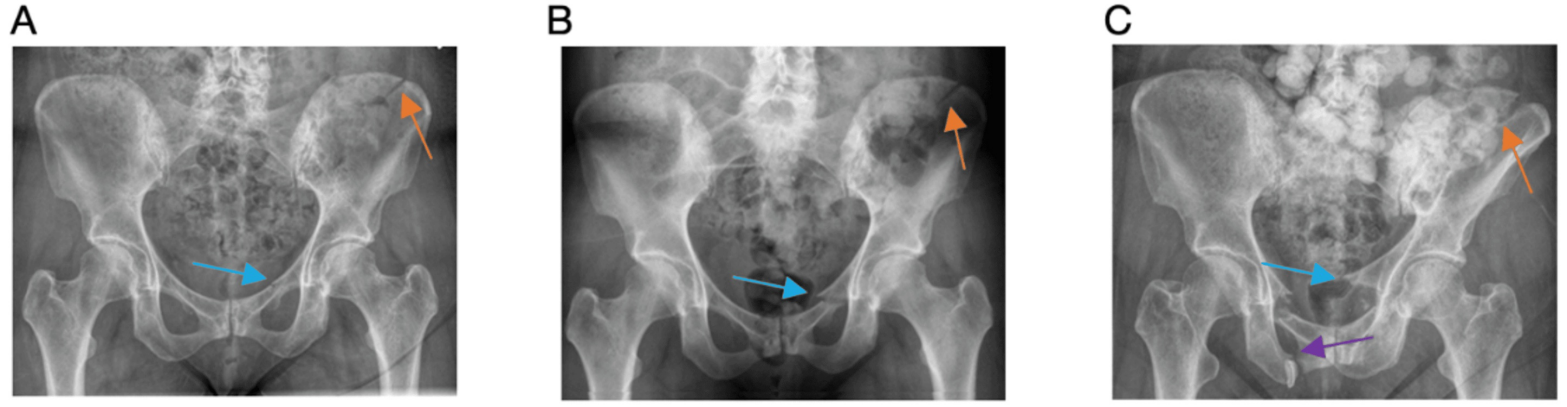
Radiographs demonstrating multiple pathologic pelvic fractures Image A shows a left superior pubic ramus fracture (blue arrow) and left iliac crest fracture (orange arrow). Image B shows more displacement of both the superior pubic ramus fracture (blue arrow) and left iliac crest fracture (orange arrow). Image C shows worsening displacement of both prior fractures of the left superior pubic ramus (blue arrow) and left iliac crest fracture (orange arrow); there is also a new right-sided superior and inferior pubic ramus fracture (purple arrow).
